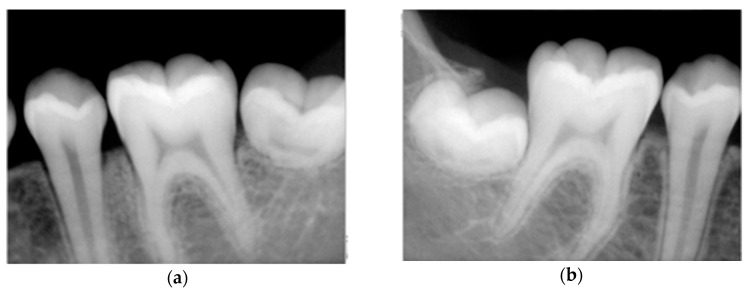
Periapical lower arch X-rays. (a) Third quadrant with 3.5, 3.6 and missing roots of 3.7; (b) Fourth quadrant with 4.5, 4.6 and missing roots of 4.7.
